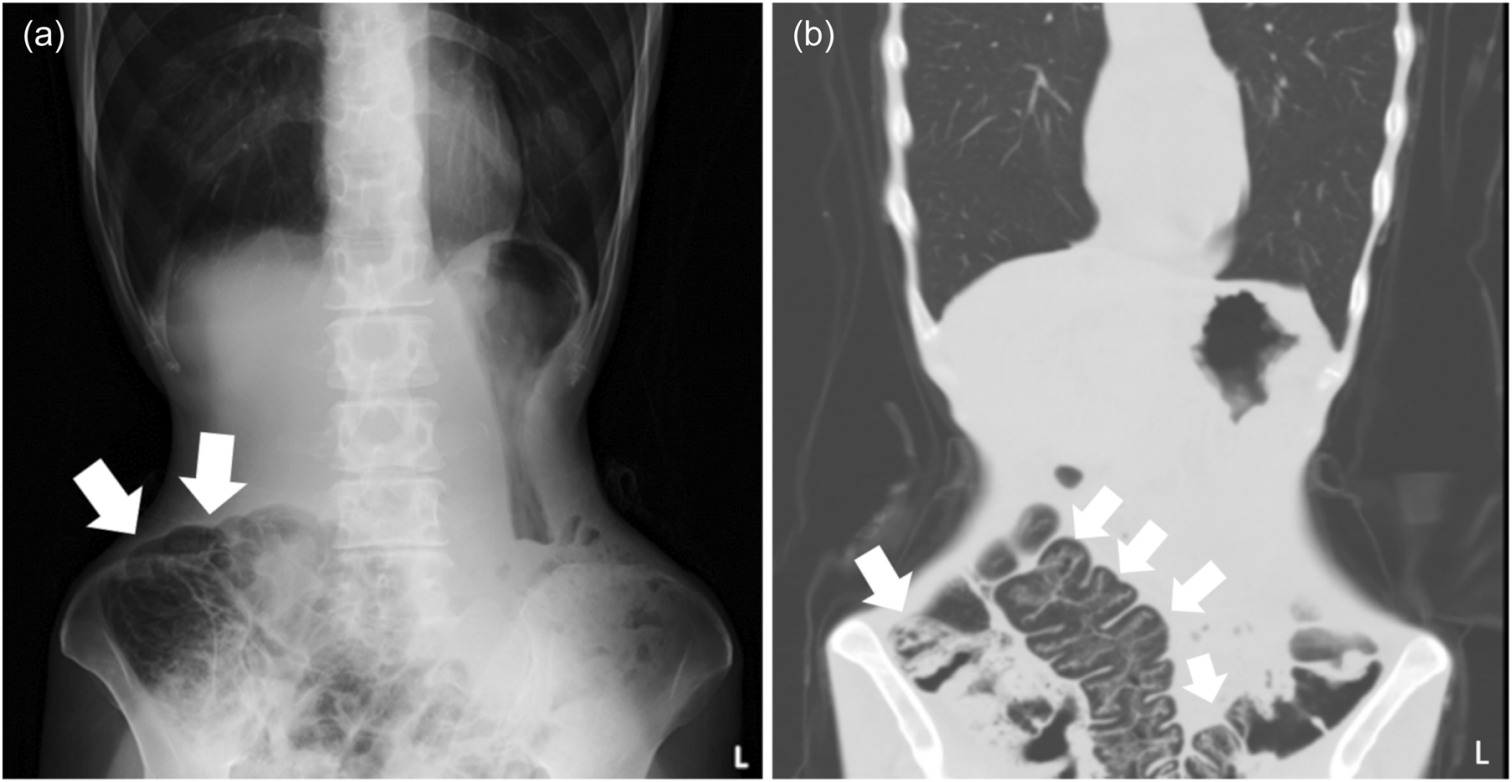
Abdominal X‐ray and abdominal CT of PCI. Abdominal X‐ray (a) shows a confluent image of grapes (arrows) and abdominal CT (b) shows cysts in the intestinal wall from the ascending colon to the transverse colon (arrows).
